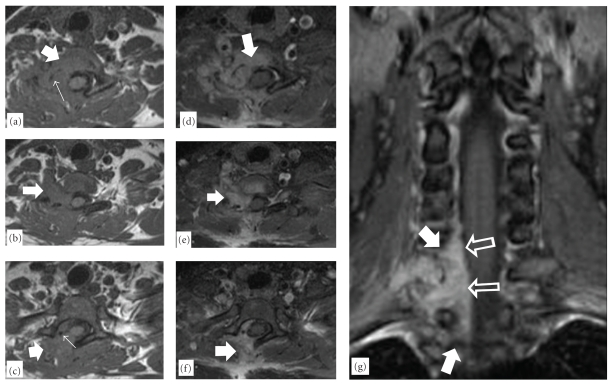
A 41-year-old female with a recurrent chondrosarcoma of the lower cervical spine. The axial T1-w MR images ((a)–(c)), show a soft-tissue mass in the right lower cervical spine (arrows) with foci of calcifications (thin arrows). The fat-suppressed contrast-enhanced MR images ((d)–(f)) show the intense enhancement of the lesion (arrows). (g) The coronal fat-suppressed contrast-enhanced MR image, shows the extension of the lesion within the right epidural space (arrows), with spinal cord displacement (open arrows).
